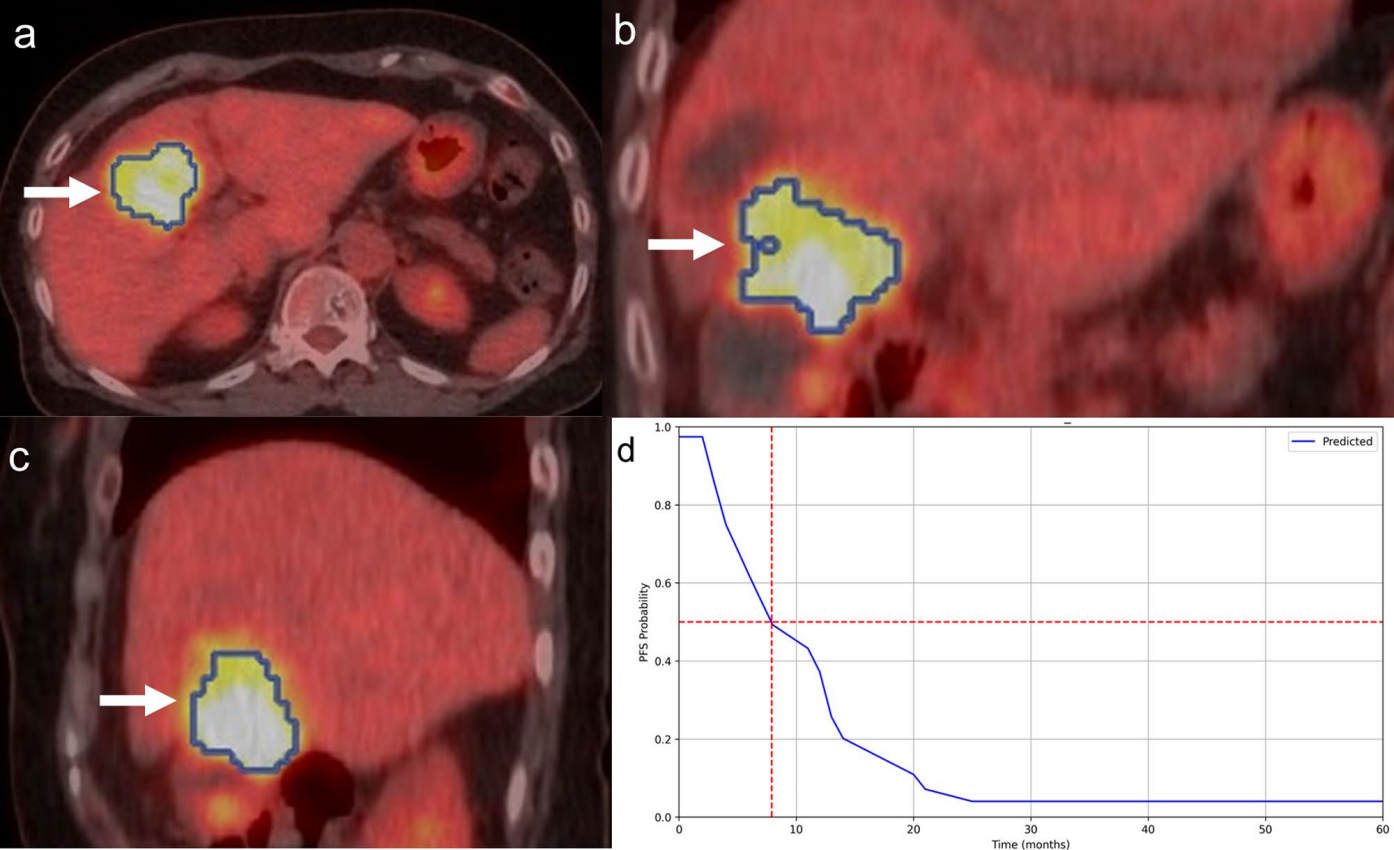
A 71-year-old woman with gallbladder cancer (adenocarcinoma, T3N1M0, stage IIIB) had progression after chemotherapy. Pretreatment [^18^F]-FDG-PET/CT scan [transaxial (a), coronal (b), and sagittal (c)] images revealed an abnormal [^18^F]-FDG uptake in the primary lesion. The blue line represents the border of the VOI (arrows). The personalized survival prediction based on the selected features ML model by the statistical feature selection method with the RSF algorithm (the best performing ML algorithm) is presented (d). The X-axis represents survival time in months, and the Y-axis indicates PFS probability. The 50% PFS probability was at 7.8 months (dotted line, d). Although the patient received chemotherapy, she developed progressive disease 4 months after therapy initiation. The patient’s 50% PFS probability was almost close to 4 months, and the ML algorithm correctly predicted the progression
